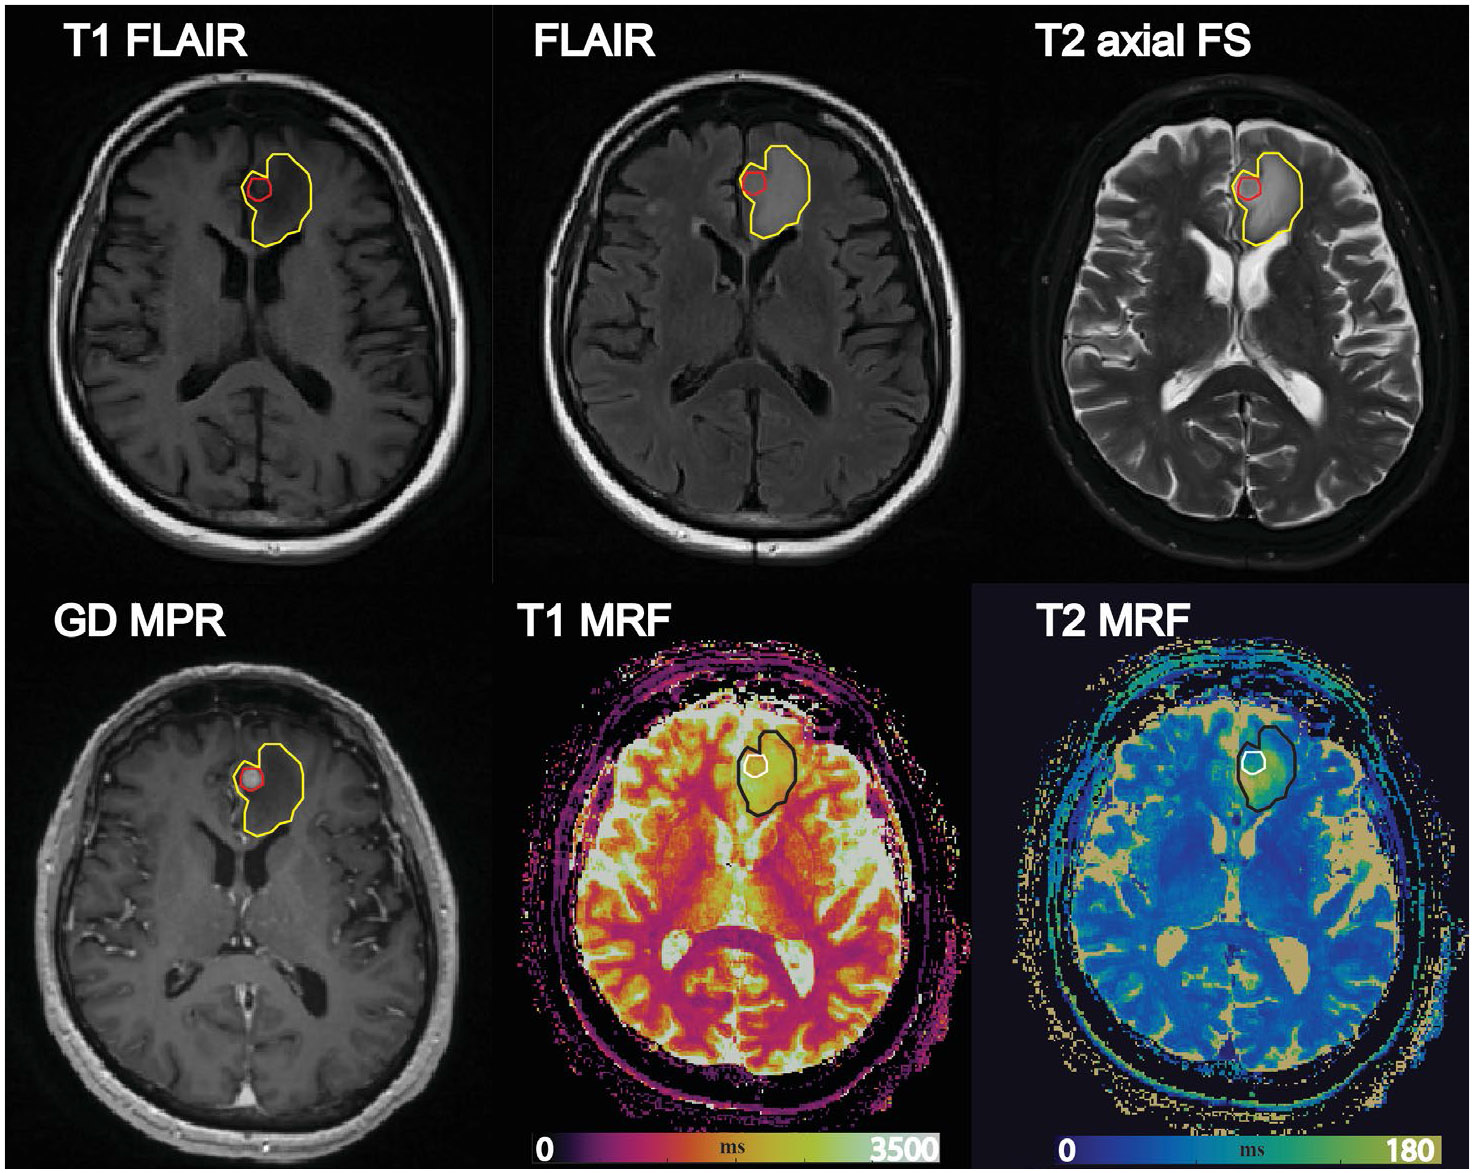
Representative results obtained from a 73-year-old male patient with a metastatic gastric tumor in the frontal cortex. MRFDK T_1_ (bottom middle) and T_2_ (bottom right) maps are shown together with pre-contrast T1-weighted, T2-weighted, and FLAIR, and post-contrast T1-weighted images. The tumor and surrounding edema are contoured for MRFDK maps and weighted images.
